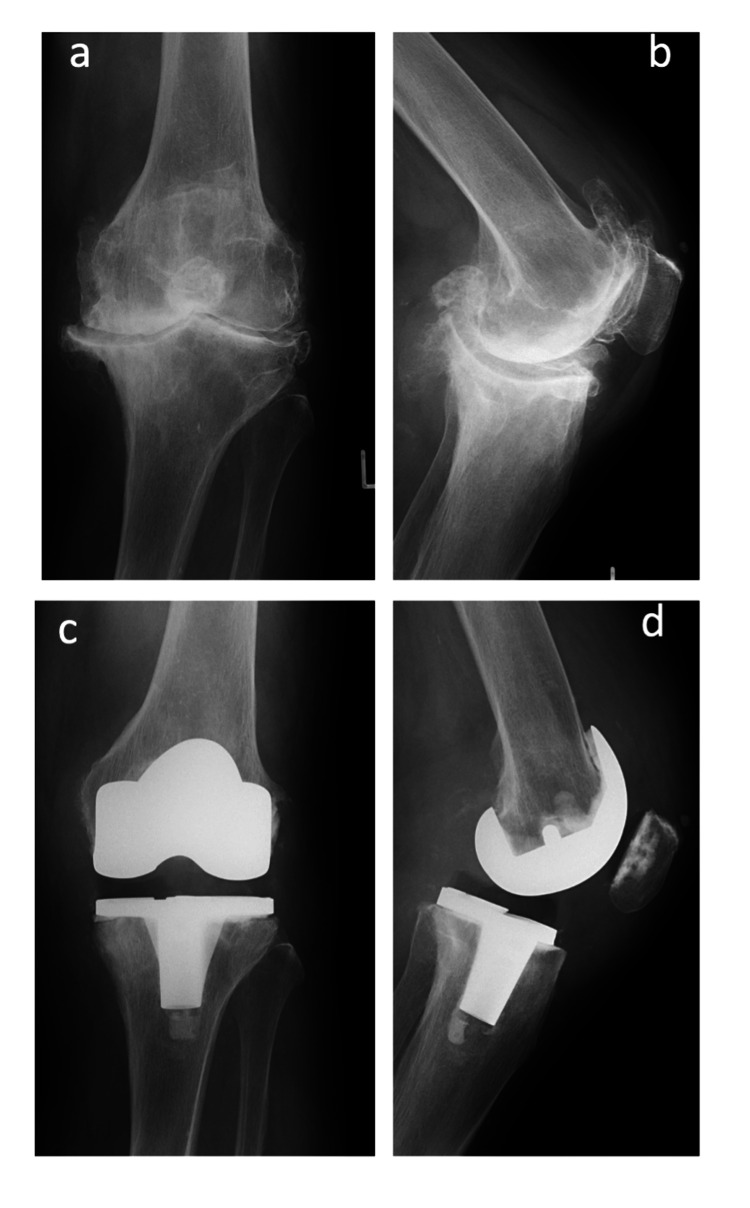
Knee radiographs before the fall. Preoperative anteroposterior (a) and lateral (b) radiographs of the left knee showed end-stage knee osteoarthritis. Anteroposterior (c) and lateral (d) radiographs after primary total knee arthroplasty.
